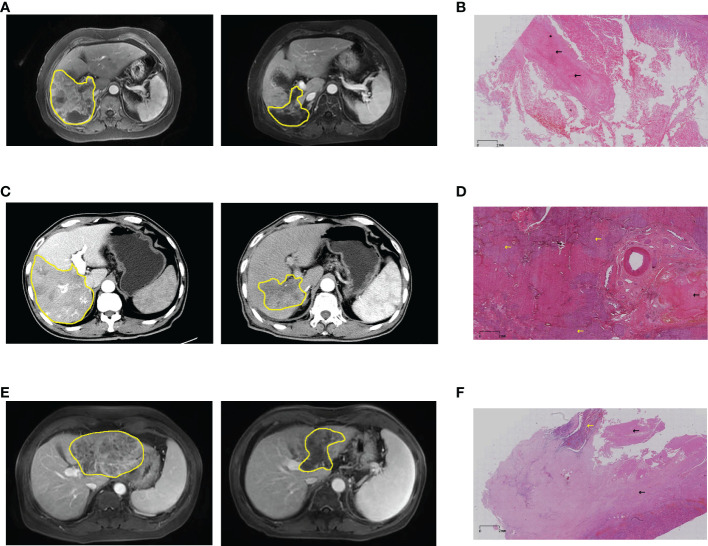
Imaging and pathological information of patients who underwent hepatectomy. (A) Pre- and post-treatment MRI of patient #8, and (B) post-operative pathology showed pathological complete response. (C) Pre- and post-treatment MRI of patient #11, and (D) postoperative pathology showed partial necrosis. (E) Pre- and post-treatment MRI of patient #18, and (F) postoperative pathology showed a large area of coagulative necrosis (80%). Yellow lines and arrows for hepatocellular carcinoma, black arrows for coagulative necrosis. MRI, magnetic resonance imaging.
